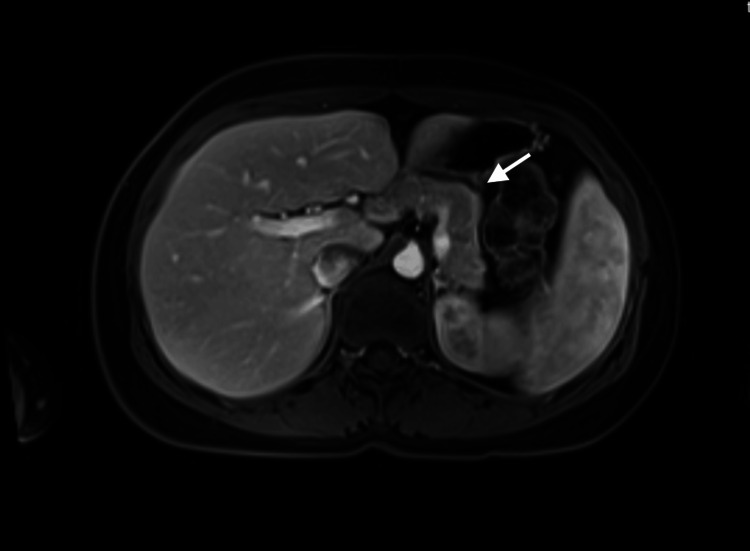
Contrast-enhanced axial MRI of the abdomen displaying normal findings of the pancreas. Axial contrast-enhanced MRI of the abdomen demonstrating normal pancreatic morphology without focal lesions, helping exclude insulinoma.
